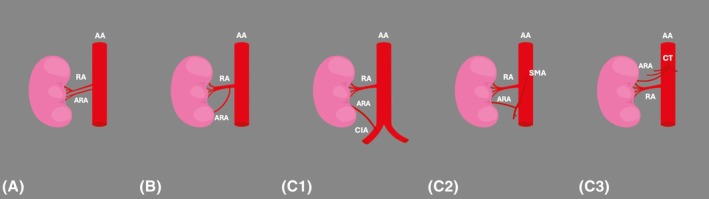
Origins of the accessory renal arteries (ARAs): (A) from the abdominal aorta (AA), (B) from the typical single renal artery (RA), (C) from other vessels, such as the common iliac artery (CIA) (C1), the superior mesenteric artery (SMA) (C2), and the celiac trunk (CT) (C3).
